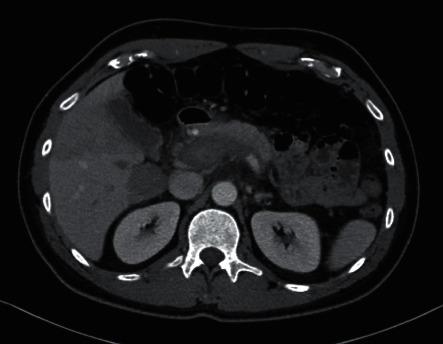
CT scan of abdomen/pelvis showing portal vein thrombosis.
